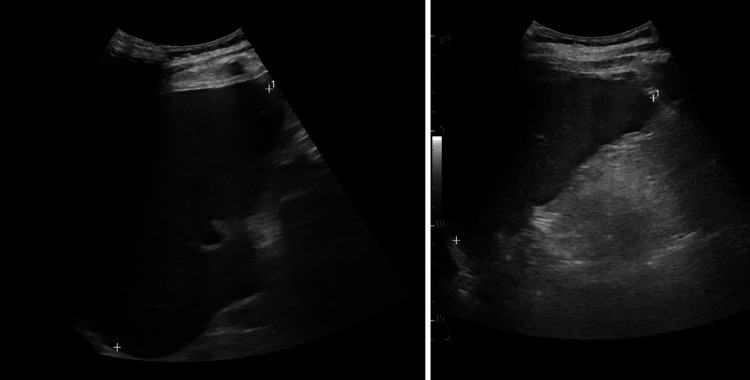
Abdominal ultrasound showing hepatomegaly on the left and splenomegaly on the right
